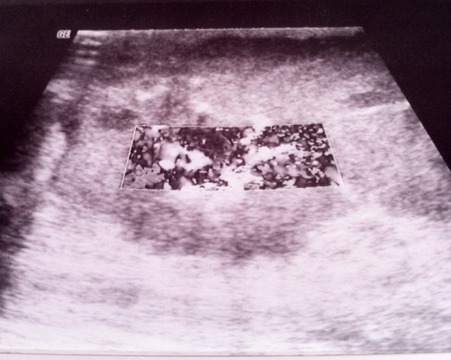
La masse est hypervascularisée au Doppler
